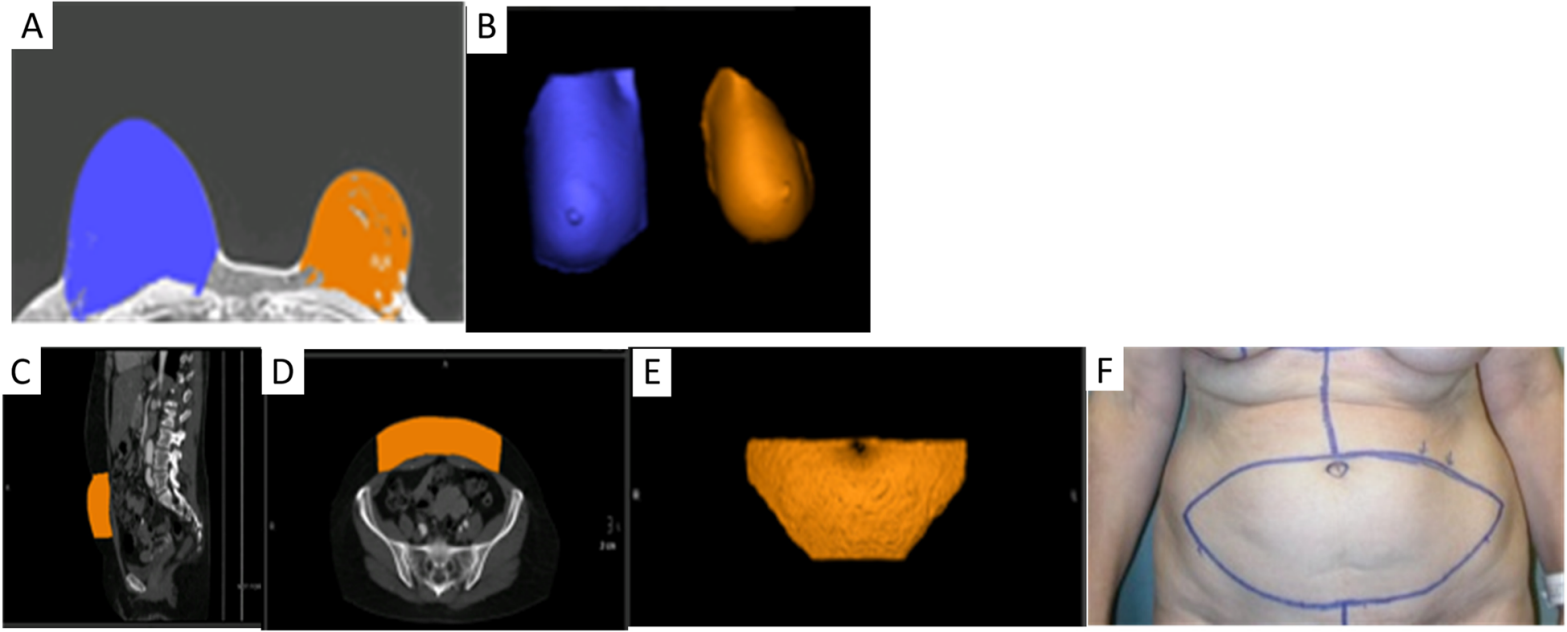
a Axial contrast-enhanced breast MRI and b 3D volume rendering obtained from this MRI used to analyze the volume of the right (blue) and left (orange) breasts, prior to planned left breast mastectomy with subsequent abdominal flap reconstruction. The volume of abdominal flap tissue that needs to be harvested to match the volume of the contralateral right breast was calculated and marked on the preoperative CTA as shown with representative sagittal (c) and axial (d) images. A virtual 3D model (e) was then generated which was used to mark the skin intraoperatively so that an appropriate volume of abdominal flap tissue can be harvested
